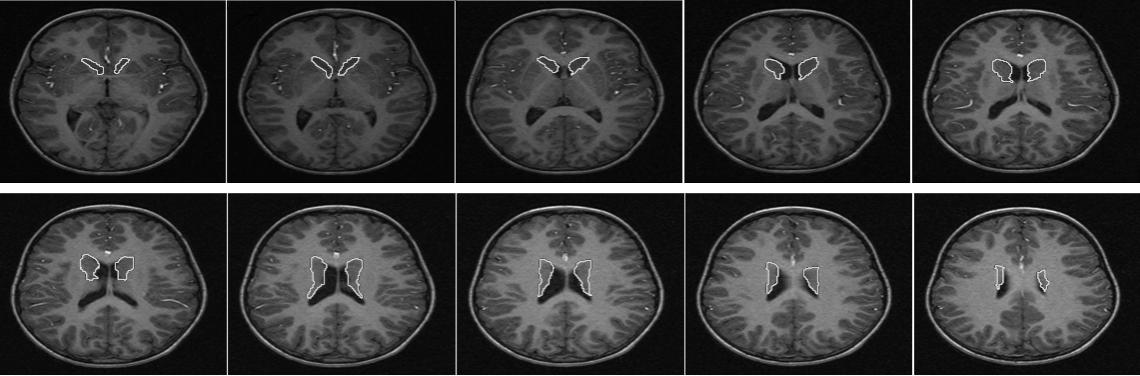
Caudate nucleus in MRI transversal planes. Examples of brain MRI transversal scans. Caudate nuclei are marked in white.
